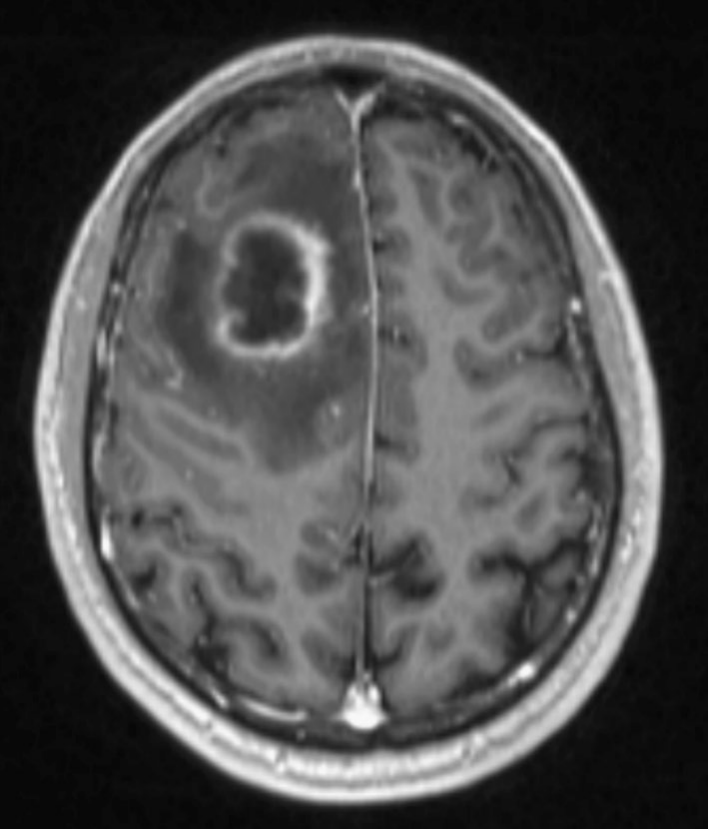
A brain magnetic resonance imaging (MRI) showing a unique brain abscess (27 × 36 × 43 mm)
